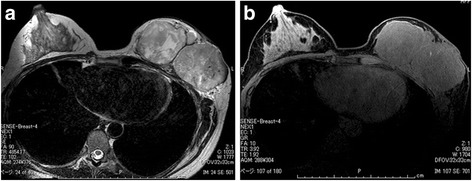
Magnetic resonance imaging findings. a The stroma shows high signal intensity on a T2-weighted image. b Magnetic resonance imaging scan showing a large tumor that was well-defined but attached to the greater pectoral muscle
